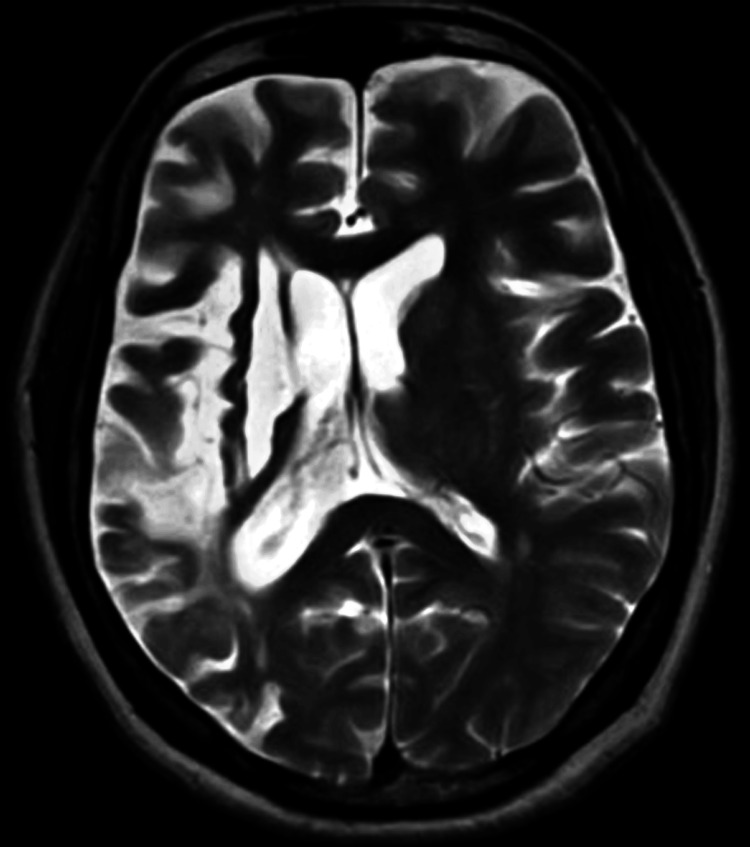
Magnetic resonance imaging (MRI) of the brain indicated an acute infarct in the right fronto-parietal-temporal lobe and gangliocapsular region (right middle cerebral artery (MCA) territory)
